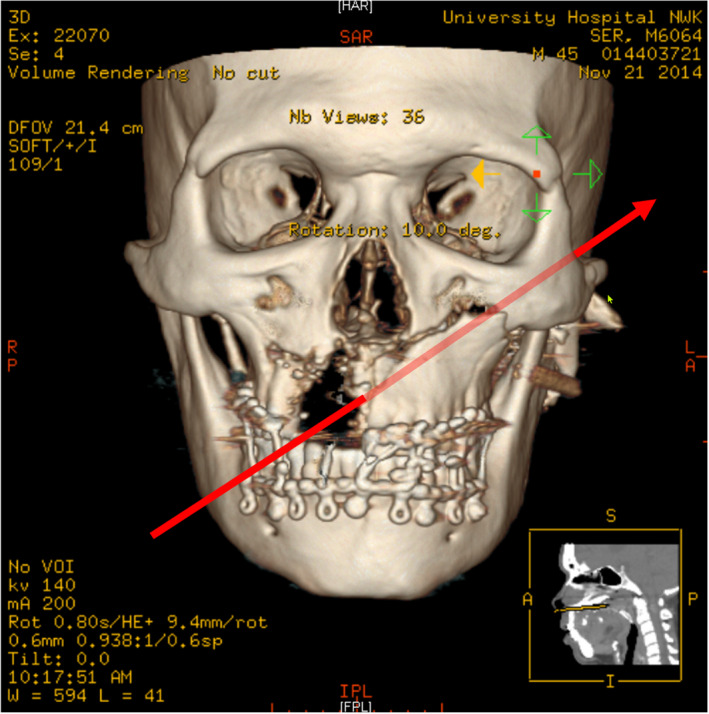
A‐P CBCT view of the entry wound. Note bullet trajectory and potential for trigeminal and facial nerve at *the site of the exit wound*
